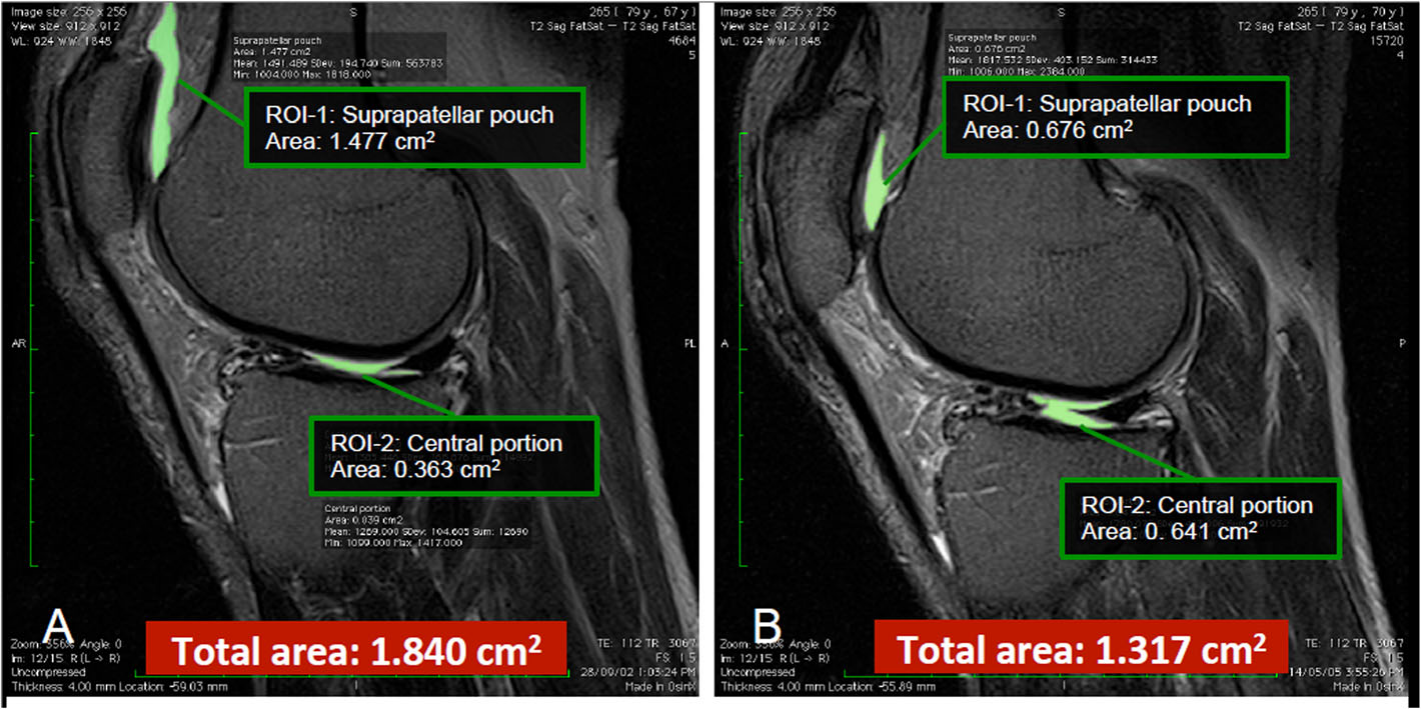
Example of changes in effusion-synovitis area (cm^2^). Effusion-synovitis size decreased from baseline to follow-up 2.6 years later (a, b). Effusion/synovitis are present in both the suprapatellar pouch and central portion. Overall, effusion size is smaller at follow-up MRI
